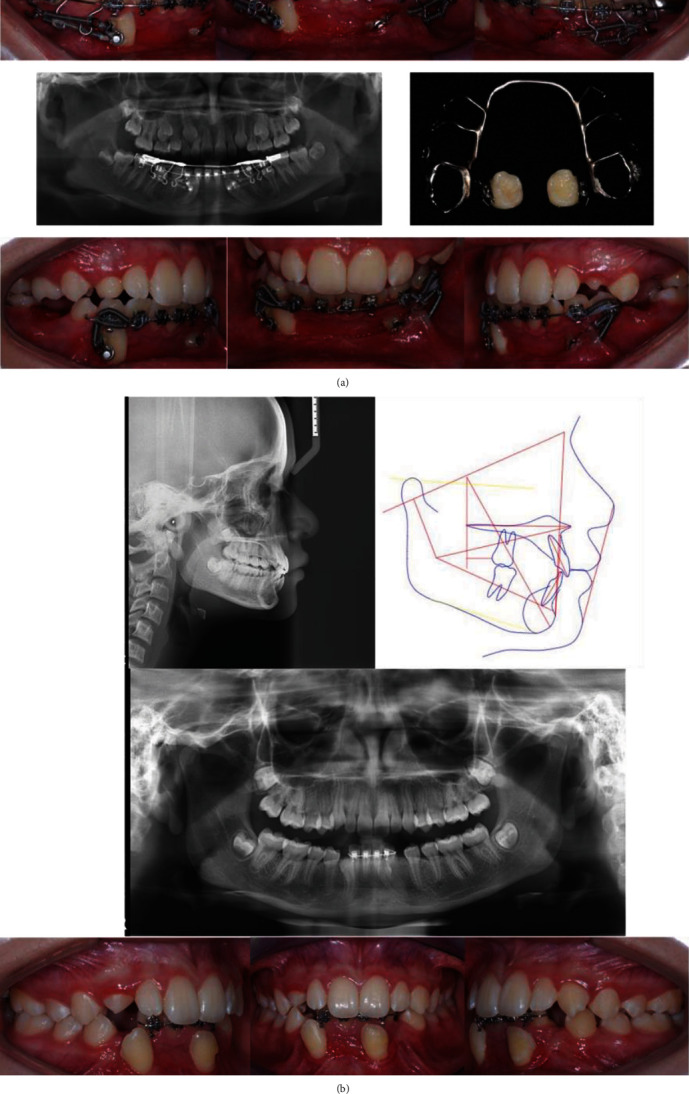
(a) Images of the first phase. Radiographic and clinical records. (b) Records of the start of the third phase of treatment.
